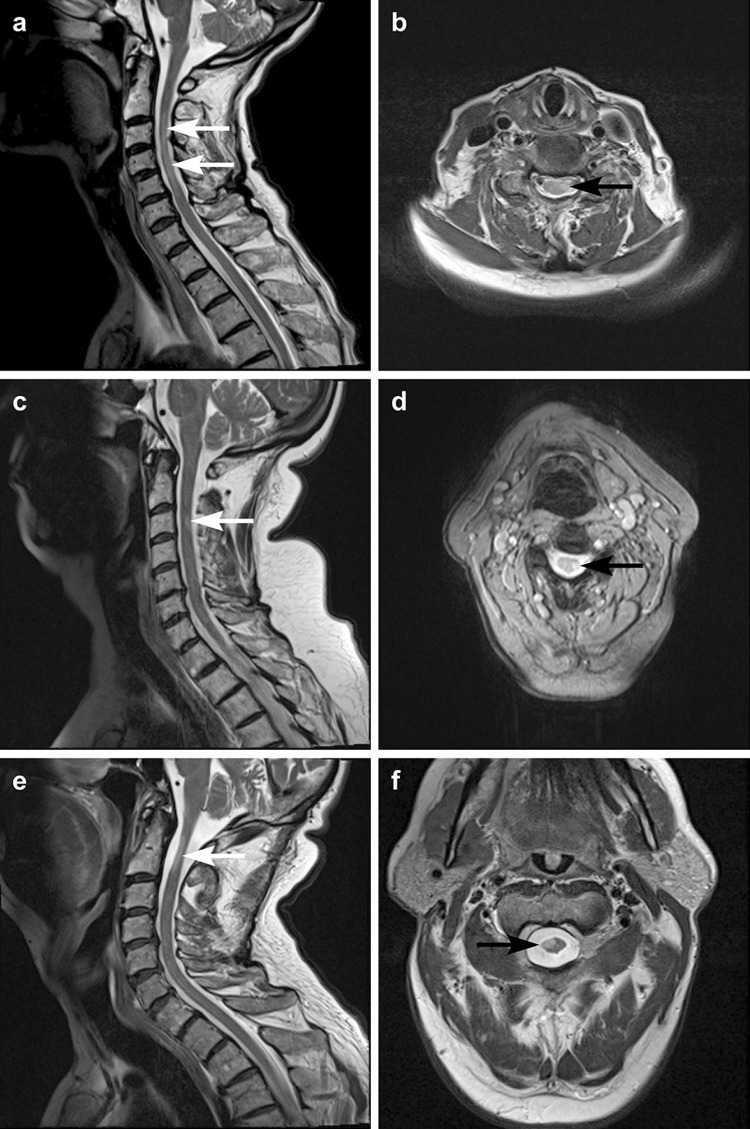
MRI findings: sagittal and axial T2 weighted images of the three patients. a, b Patient (1) T2 hyper-intensity in the left lateral aspect of the cervical cord at C3–C5 vertebral levels. c, d Patient (2) focal T2 hyper-intensity involving the left lateral aspect of the cord at C3/4 level. e, f Patient (3) T2 hyper-intensity involving the right anterolateral aspect of the cord at C1/2 level. All three cases have signal abnormality involving the cervical cord which is eccentric in location and involves more than one vertebral level in length
